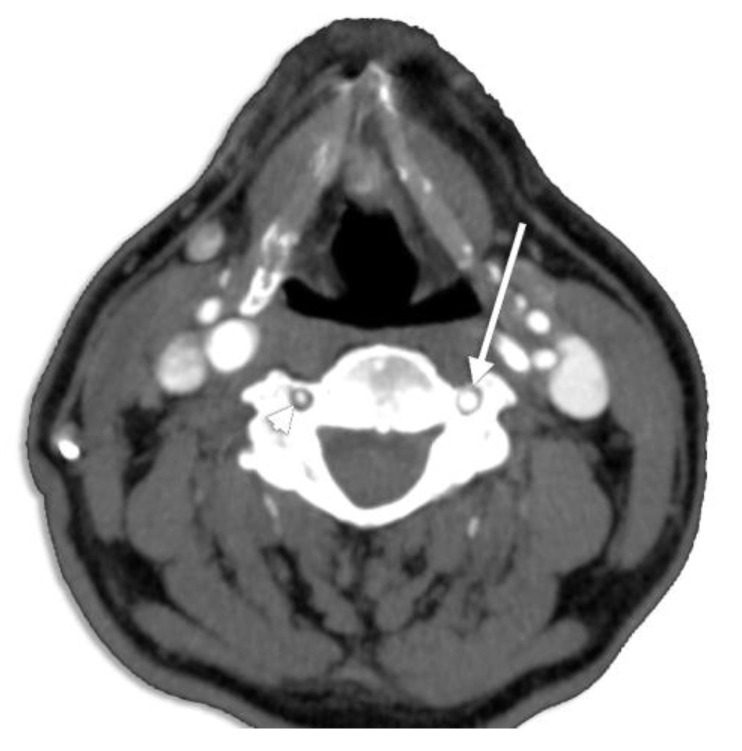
Axial CT slice at the level of C4 viewed inferiorly. Right vertebral artery hypoplasia (arrowhead). Normoplastic left vertebral artery (arrow).
